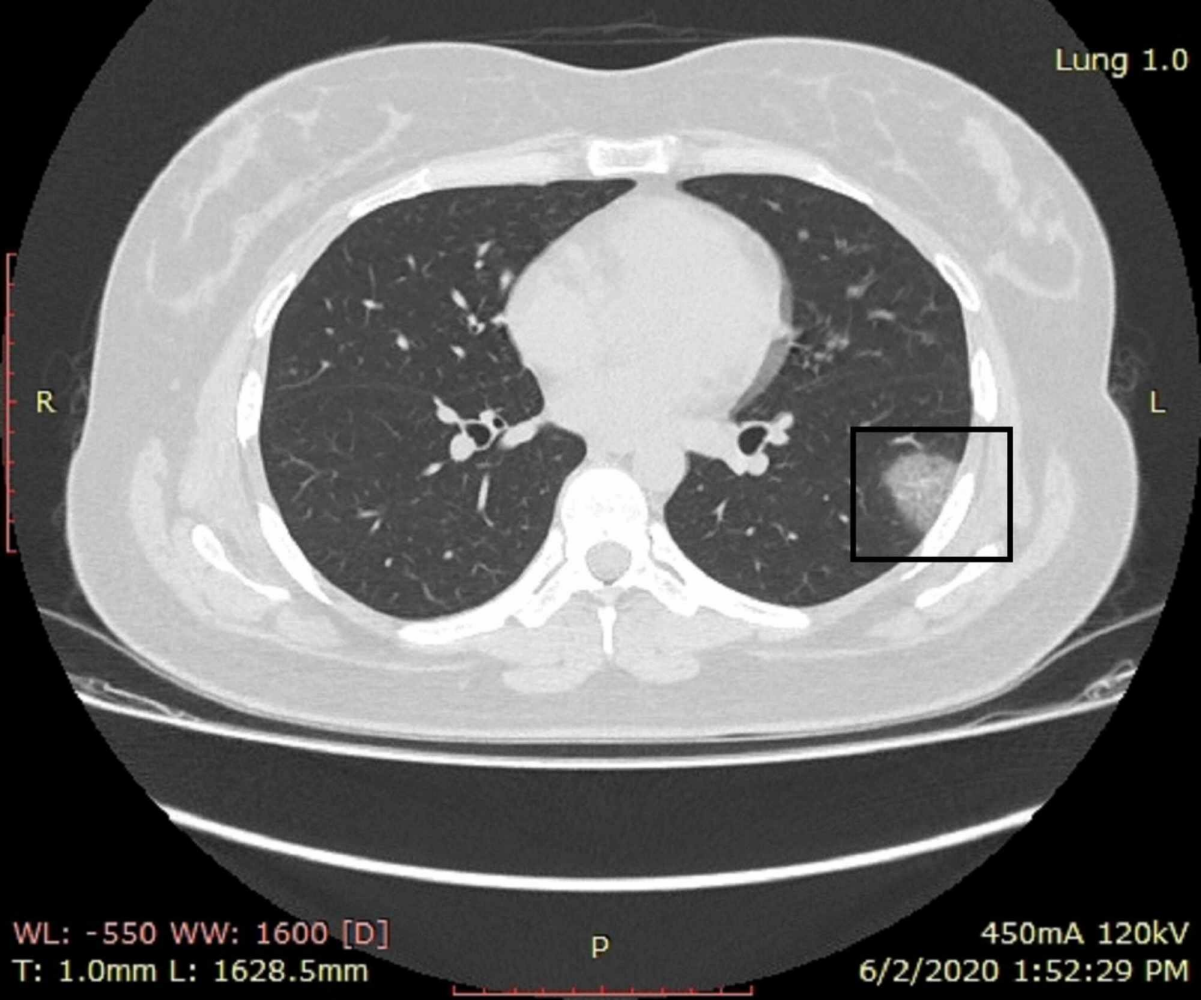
A 30-year-old female patient with mild symptoms CT shows a single patch of ground-glass opacity in the superior segment of the left lower lobe (box) CT: computed tomography
